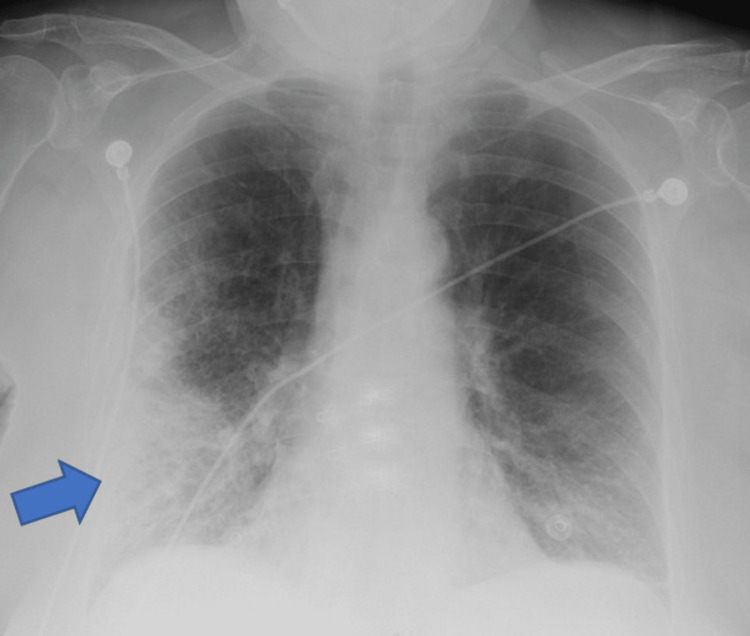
Patient's admission chest X-ray. Blue arrow pointing at pneumonia in the right lower lobe
